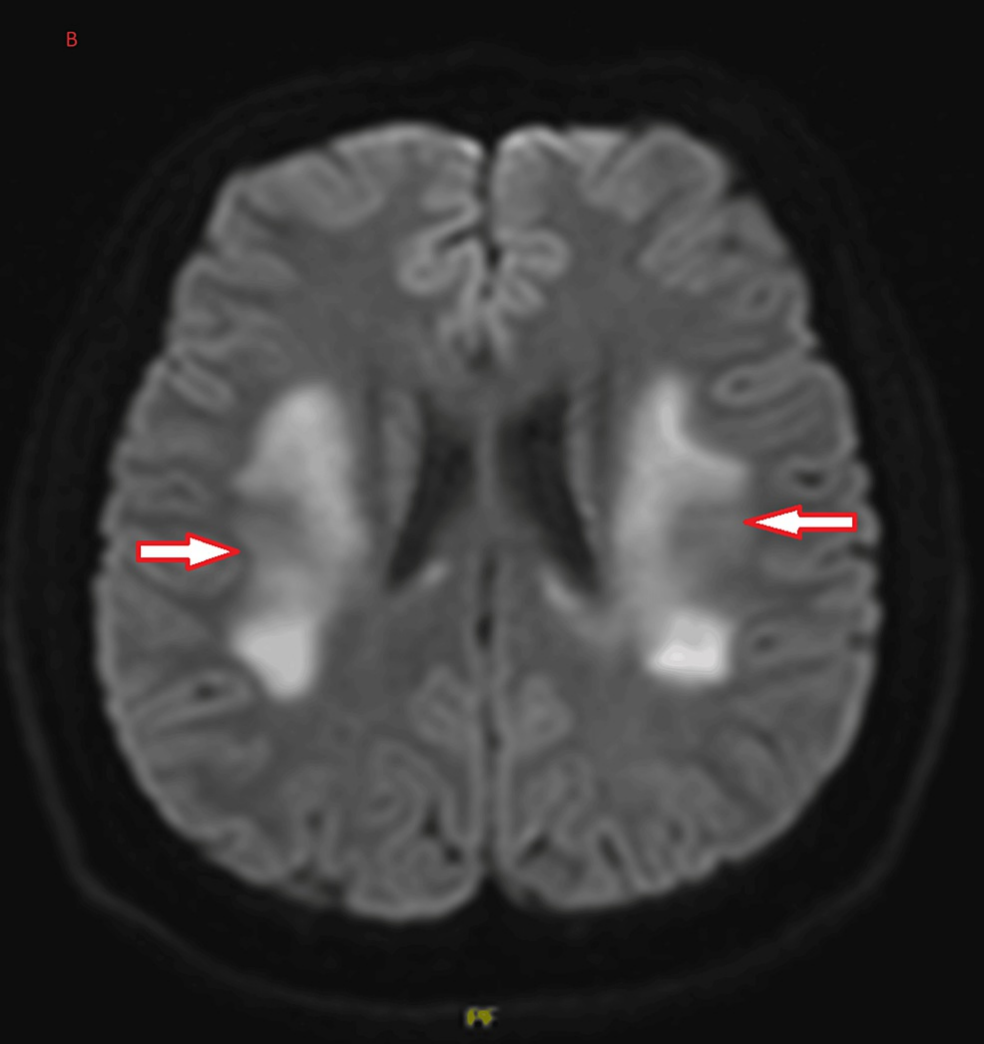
DWI hyperintense lesions. The diffusion-weighted imaging (DWI) showed hyperintense lesions, as indicated by the arrows.
